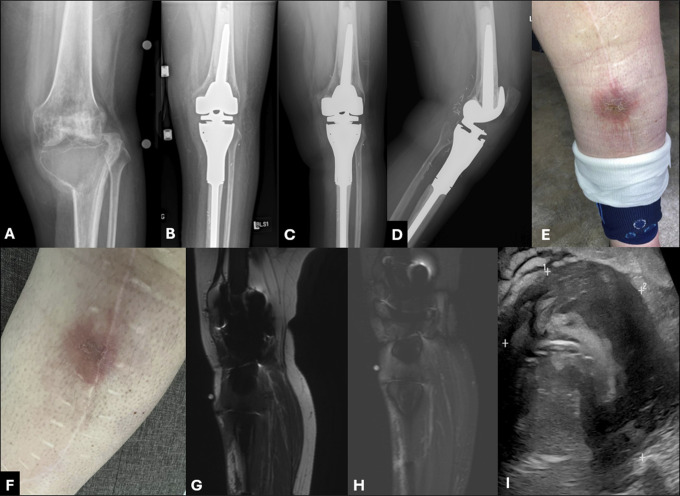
Preoperative AP radiographs and images showing a pathologic fracture of the proximal tibia through a large lytic lesion (A) with pathology showing giant cell tumor of bone. The patient was treated with a cemented proximal tibia endoprosthesis (B) and was doing well for 3 years. At 3 years postoperatively, the patient called in with concerns of their surgical incision. Radiographs were repeated (C and D) and showed no signs of implant loosening. Laboratory studies were negative for an acute infection; however, the patient developed a wound over the incision (E). They were treated empirically with oral antibiotics locally for a concern for a cellulitis. The erythema decreased; however, the wound persisted (F). Metal-suppression MRI with and without contrast was performed and on sagittal T1 (G) and STIR (H) images showed no signs of an abscess. However, owing to the limitations of metal-suppression MRI, an ultrasonography was recommended. An ultrasonography showed no fluid collection to suggest an abscess; however, there was a hypoechoic mass (I).
